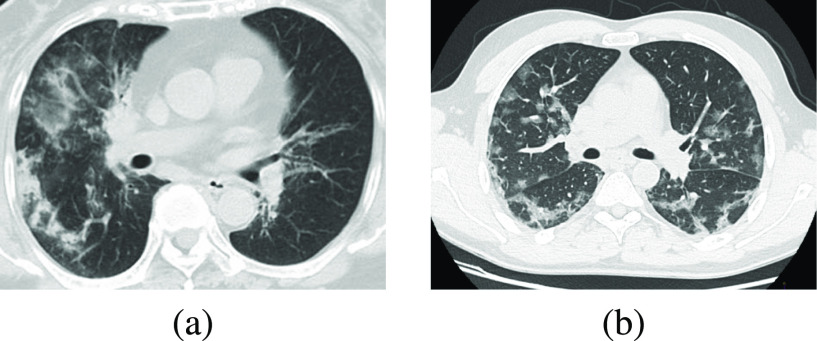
Examples of (a) non-COVID-19 and (b) COVID-19 positive CT scans.
